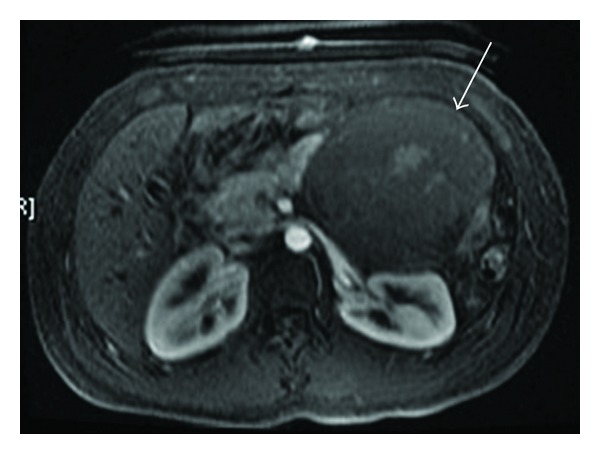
SPEN (arrow) with wall, internal septations and hemorrhage on MRI.
